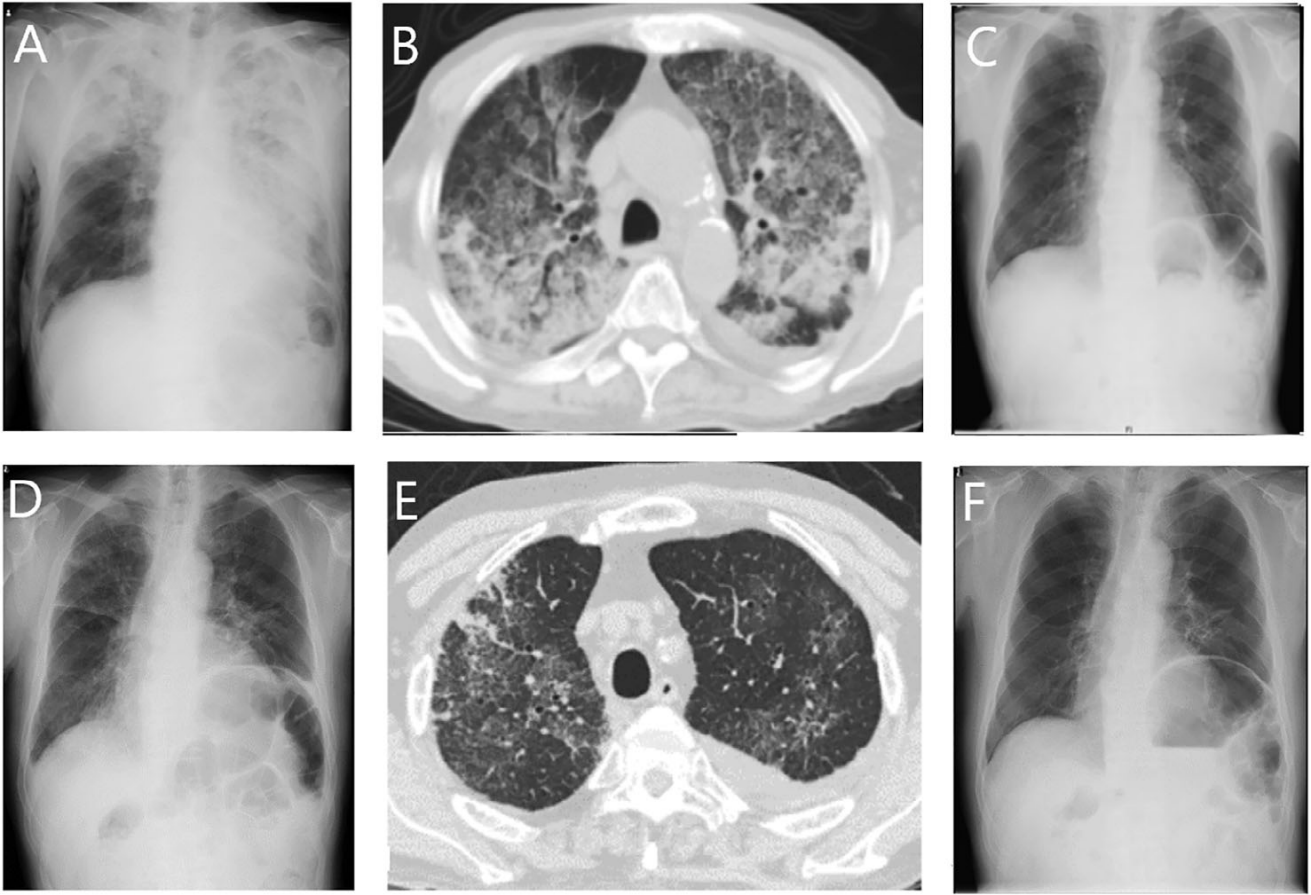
(A) Chest radiograph after the patient's hospitalization revealed a consolidation in the left lung and right upper lung. (B) Computed tomography on hospitalization showed a mixed pattern of air‐space consolidation, air bronchogram, and ground‐glass opacities. (C) Chest radiograph at discharge showed improvement in consolidation. (D) Chest radiograph at readmission showed ground‐glass opacities and pleural effusion in both lungs. (E) Computed tomography at readmission also showed ground‐glass opacities and pleural effusion in both lungs. (F) Chest radiograph after benralizumab therapy showed improvement of ground‐glass opacities and pleural effusion.
